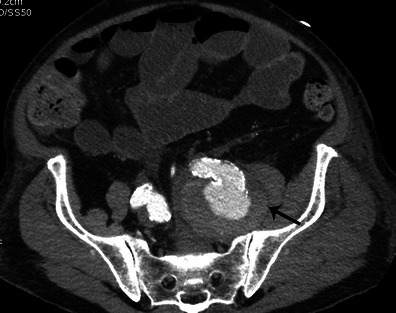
Abdominal and pelvic contrast-enhanced CT performed for the recent onset of left back pain in a patient with infective endocarditis. The axial image shows a saccular aneurysm of the ostium of the left internal iliac artery (*black arrow*)
